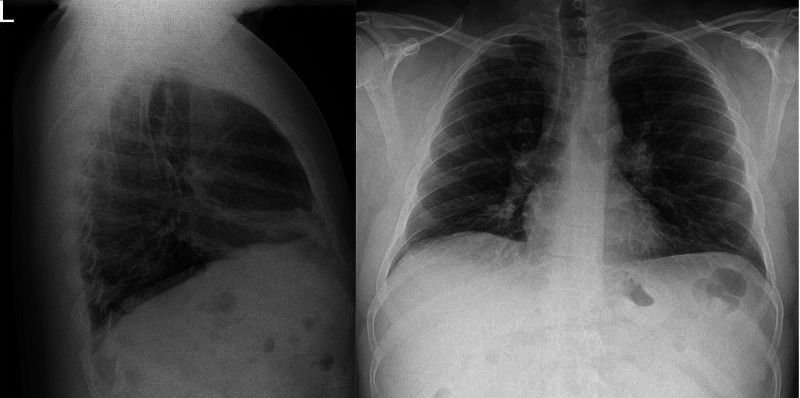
Radiographic demonstration of pulmonary infiltrates Left: lateral chest X-ray that shows that the posterior triangle has infiltrates at the level of the lingula. Right: posterior-anterior view chest X-ray did not demonstrate infiltrates
